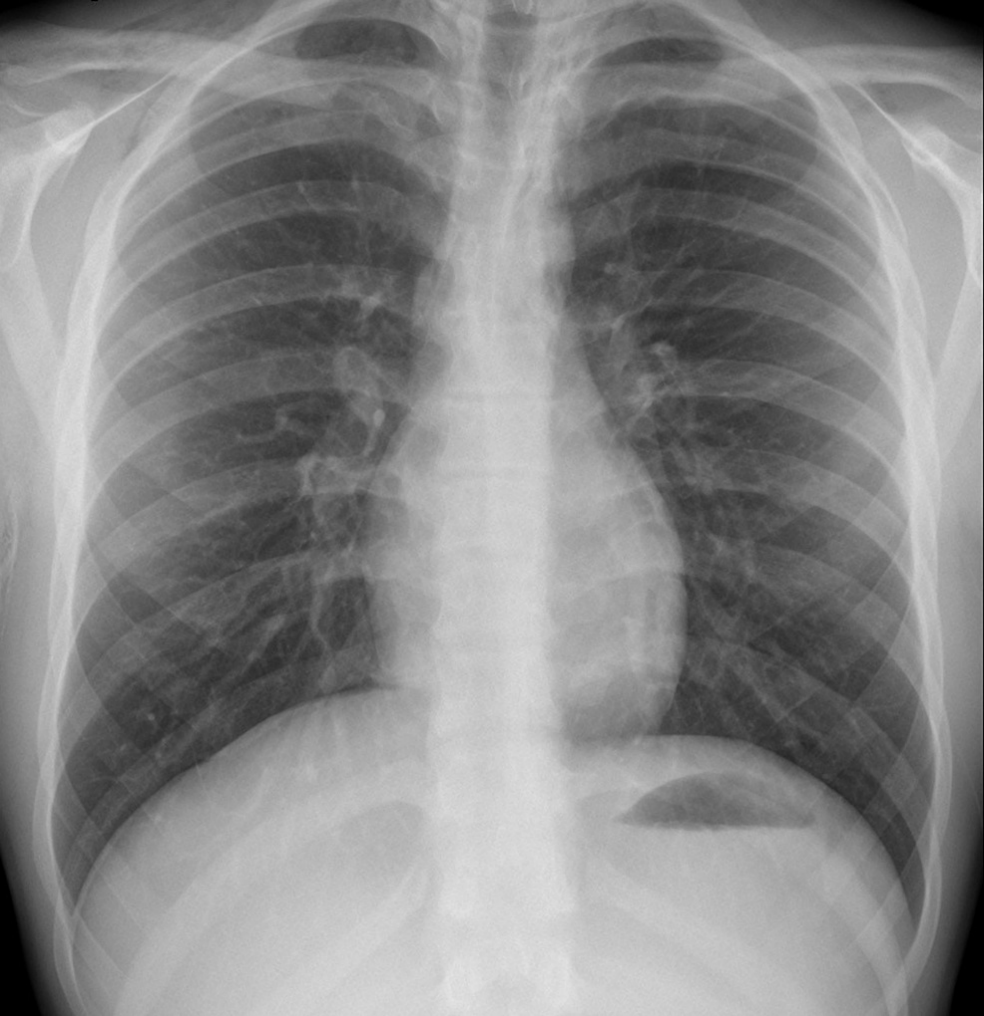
Chest X-ray on day 3 showing partial resolution of emphysema and pneumomediastinum.
